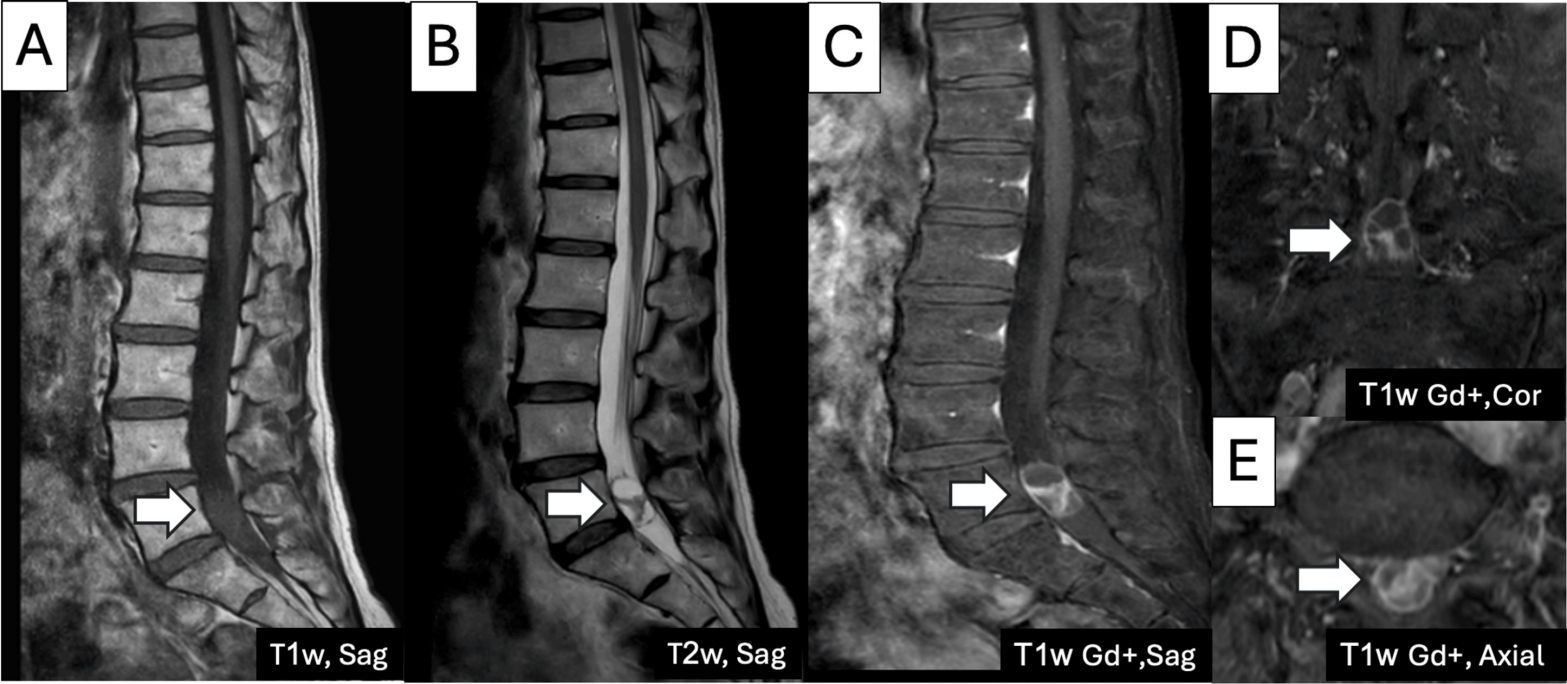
The MRI of the lumbar spine reveals a heterogeneously well-circumscribed mass at the L5 level that is iso-intense on T1-weighted (T1W) image (A) and hyper-intense on T2-weighted (T2W) image (B), enhanced after the contrast injection on the sagittal plane (C), coronal plane (D), and axial plane (E).
